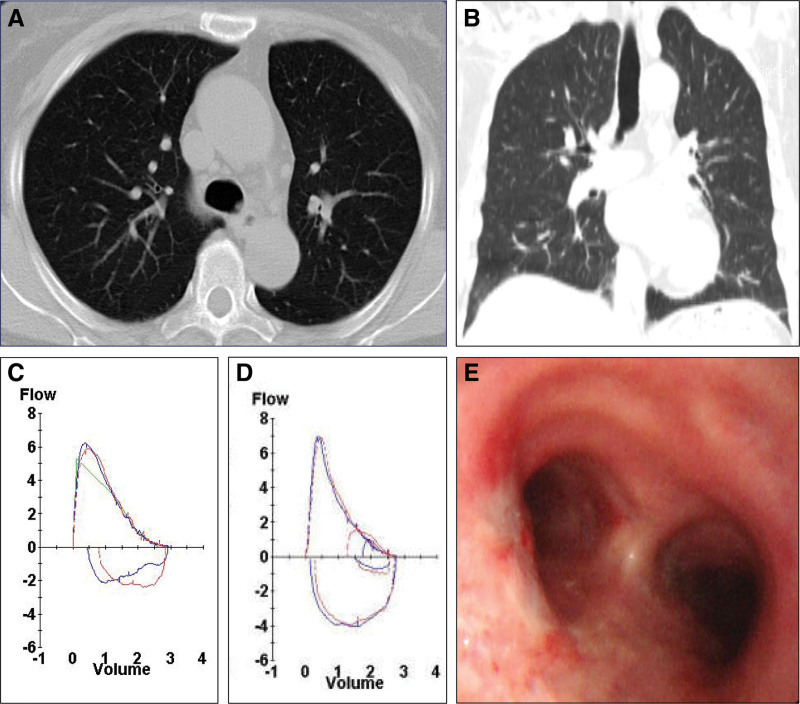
Follow-up examinations 1 mo after the completion of the treatment. Chest CT scans of the transverse (A) and coronal views (B). Flow-volume curves from the initial (C) and follow-up (D) pulmonary function tests. (E) The bronchoscopic picture showing the healing stage with fibrotic scar formation on the previous site of the endotracheal neurofibroma. CT = computed tomography.
